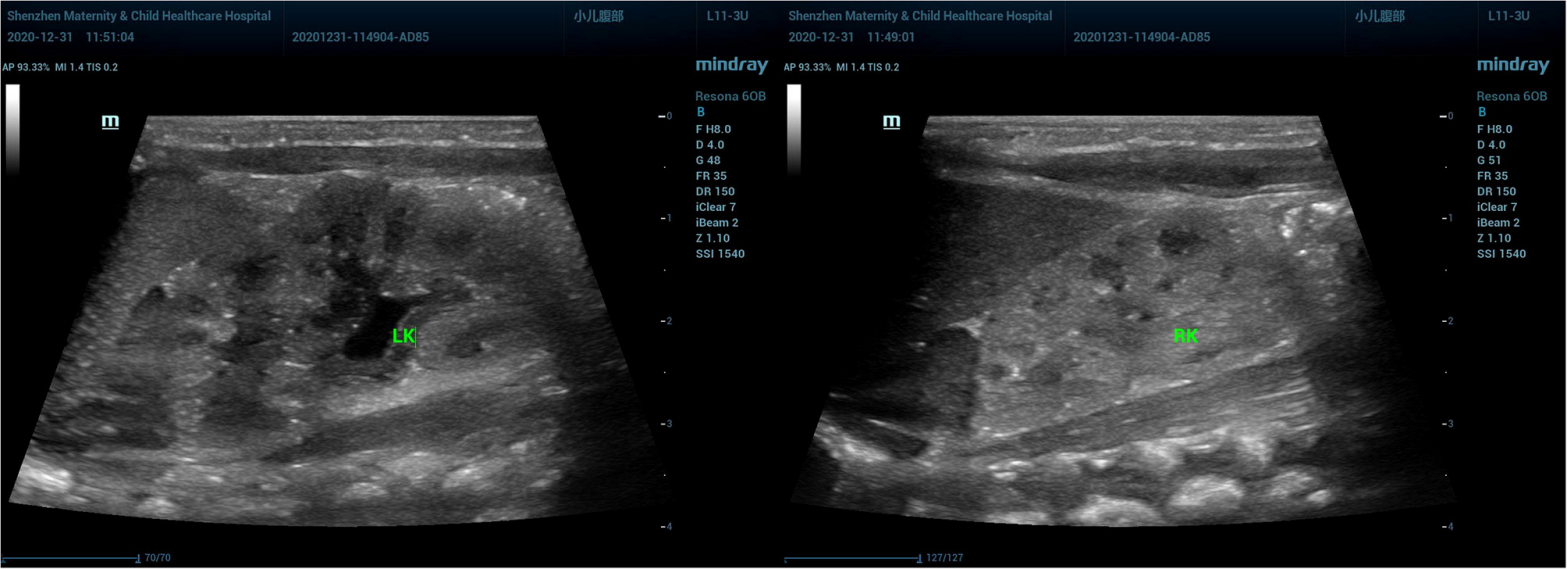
The kidney ultrasound of the male neonate. Normal kidneys and adrenal glands are shown.
